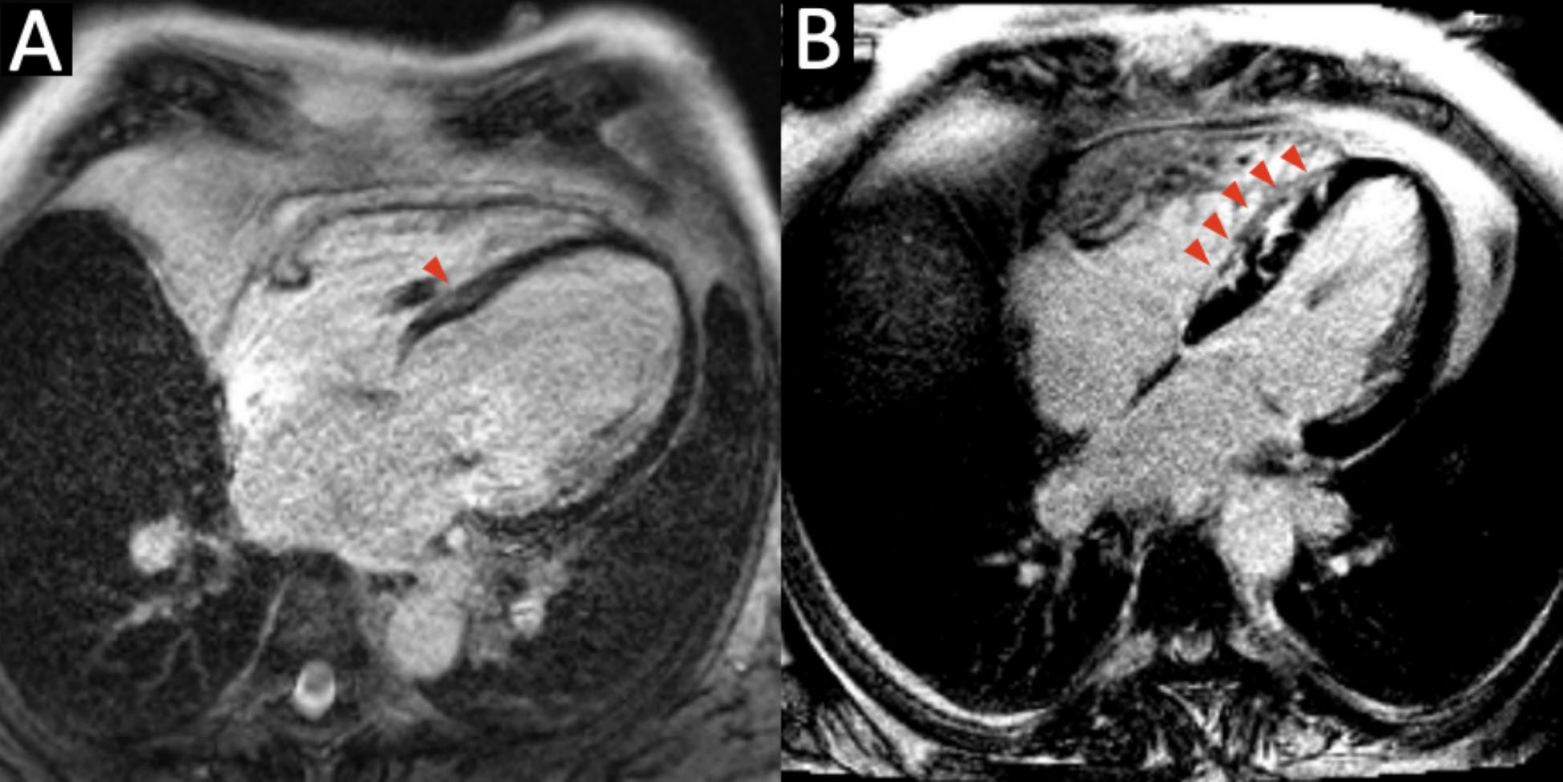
Demonstration of cardiac magnetic resonance imaging in dilated cardiomyopathy and cardiac sarcoidosis. Both panels present the four-chamber view of cardiac magnetic resonance imaging (CMR). A A patient with dilated cardiomyopathy (DCM), featuring mid-myocardial enhancement in the septum and a notably dilated left ventricle, indicated by the arrow. B A patient with cardiac sarcoidosis, highlighting multifocal late gadolinium enhancement in the interventricular septum, as indicated by the arrows
